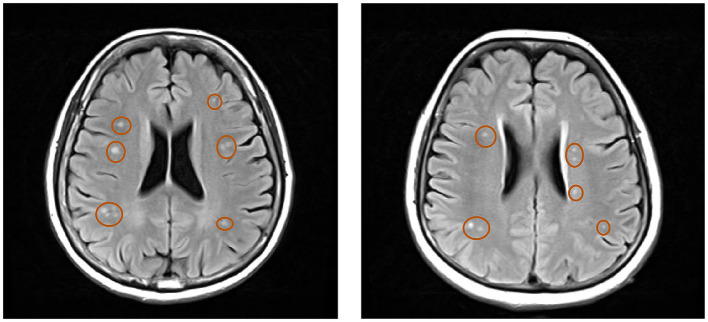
Axial T2-FLAIR MRI from two different patients with migraine. Image 1 (left) shows subcortical white matter hyperintensities (WMH) in a frontoparietal distribution. Image 2 (right) demonstrates WMH in a periventricular in addition to in a frontoparietal distribution.
